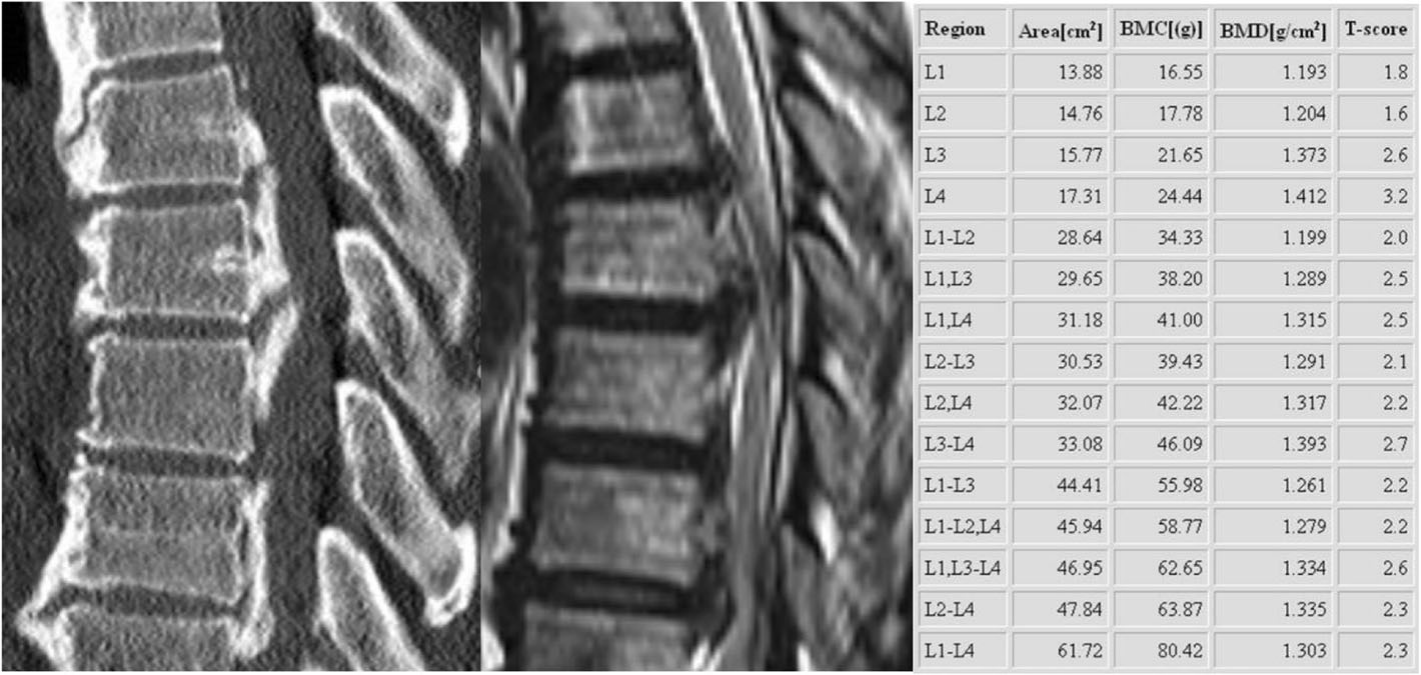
A 53-year-old woman presented with a 4-month history of gait disturbance and bilateral leg weakness (Grade 3) after falling. Computed tomography scan shows ossification of the posterior longitudinal ligament (OPLL) at T5–9. Thoracic cord compression with signal change is observed on magnetic resonance imaging. Bone mineral density with T-scores is greater than that of the same-aged healthy controls
